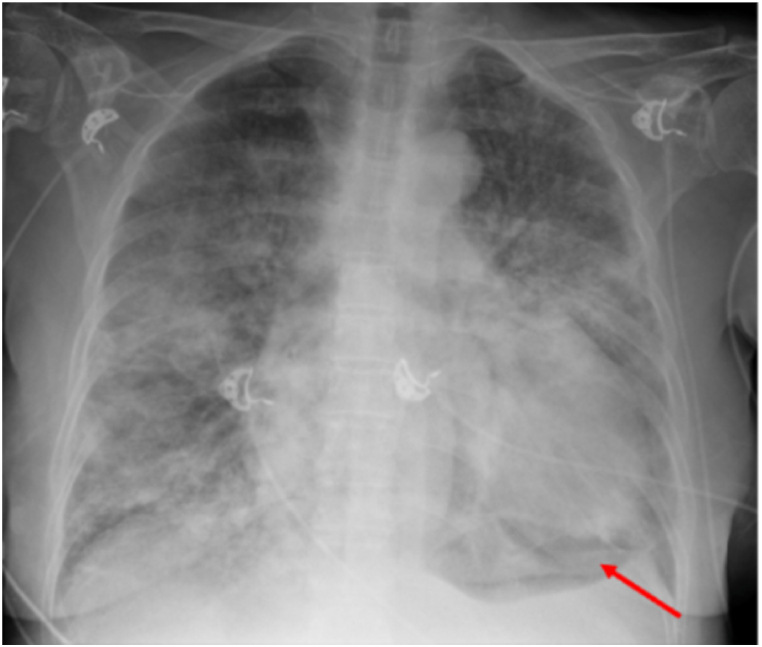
Chest X-ray performed two days after hospital admission and pericardial drainage shows an enlarged cardiac silhouette, congested pulmonary vasculature and bilateral pulmonary opacities. In addition, pneumopericardium is visible (arrow), likely secondary to the recent pericardial drainage.
